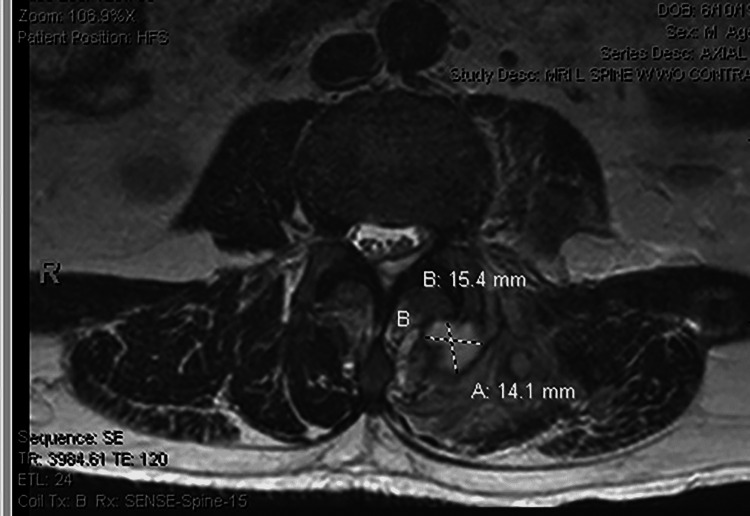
MRI (axial T2 sequence) showing multifocal left paraspinal abscesses and phlegmon, with some possible communication: T2 hyperintense left paraspinal collection at the approximate L4 level measures roughly 1.4 cm (AP) x 1.5 cm (TRV) x 2.8 cm (SI) and abuts the dorsal aspect of the left L3-L4 facet joint. AP: Anteroposterior; TRV: Transverse; SI: Superior to inferior.
